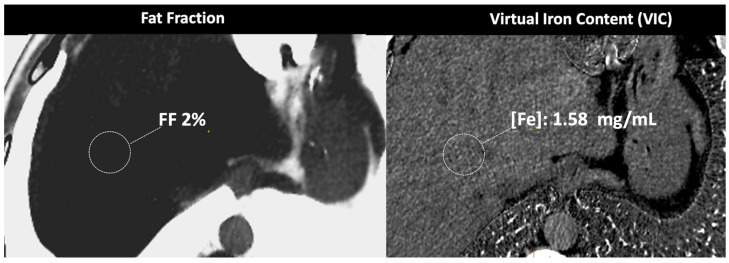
DECT-based assessment of fat and iron deposits. CT images demonstrate a fat fraction of 2% and a virtual iron concentration of 1.58 mg/mL, which rules out fatty infiltration or iron overload in this patient.
